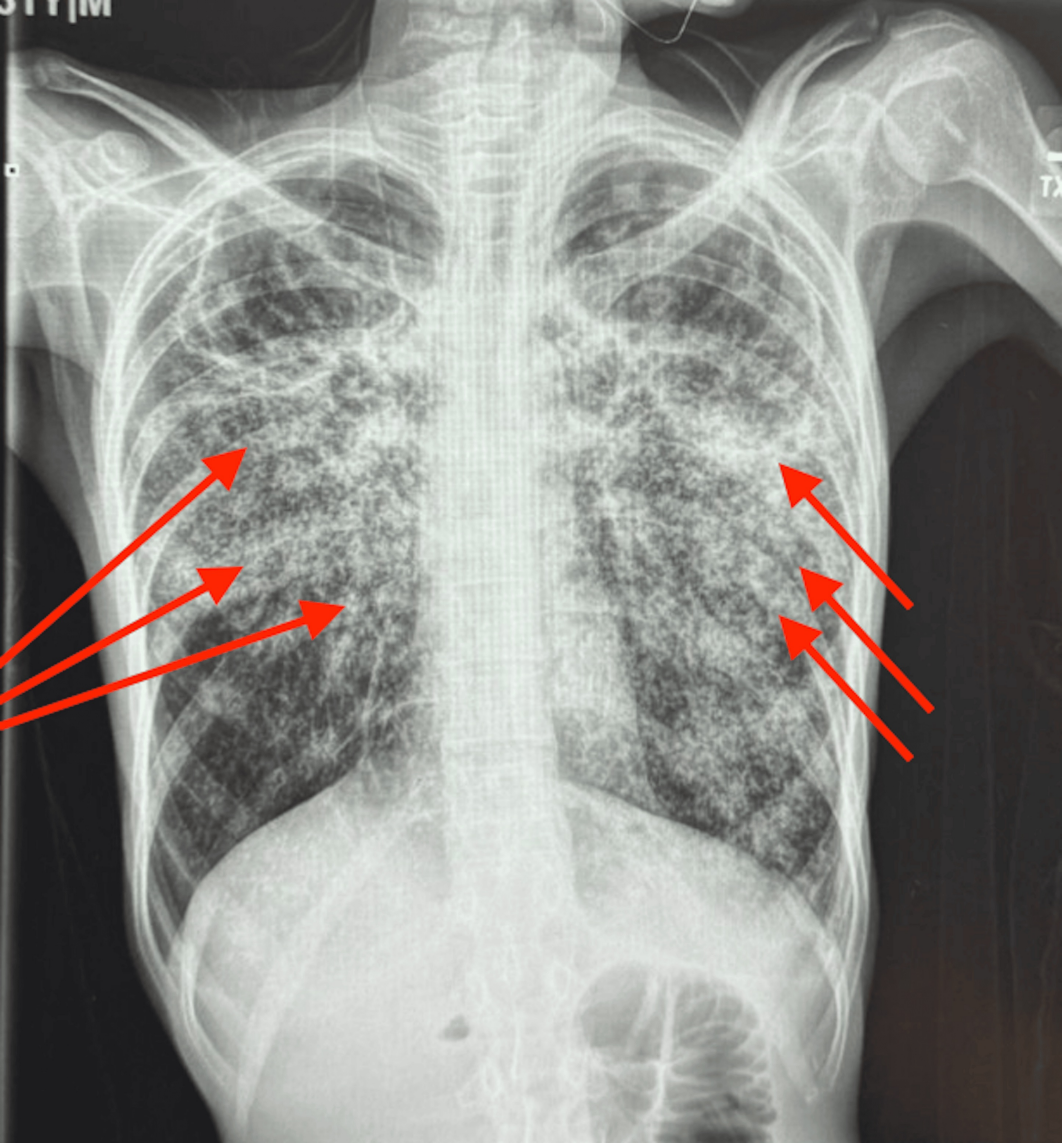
Chest X-ray (single view) showing bilateral reticulonodular infiltrates. Chest radiograph demonstrating diffuse bilateral reticulonodular and micronodular opacities throughout both lung fields (arrows), consistent with a miliary pattern of pulmonary disease.
